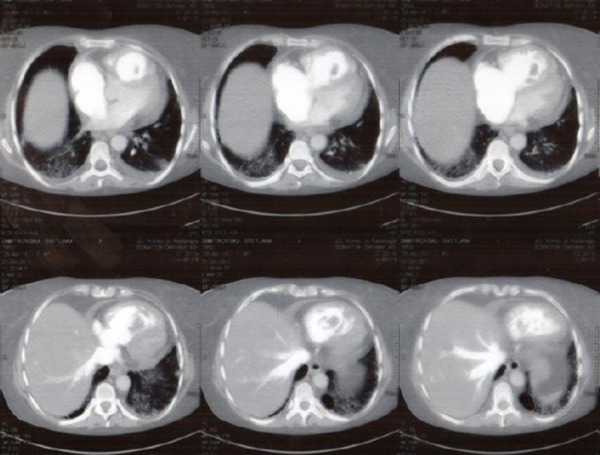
Cardiac CT showing extensive thrombotic material in right ventricle (RV), small bilateral pleural effusions, and tiny layer of pericardial effusion*.
